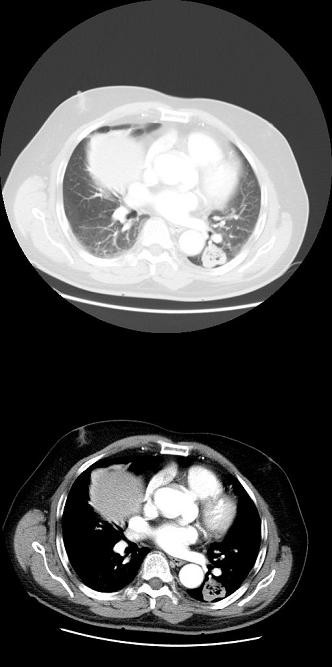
Thoracic CT-scan: Peripheral mass in left lower lobe with central cavitation.
